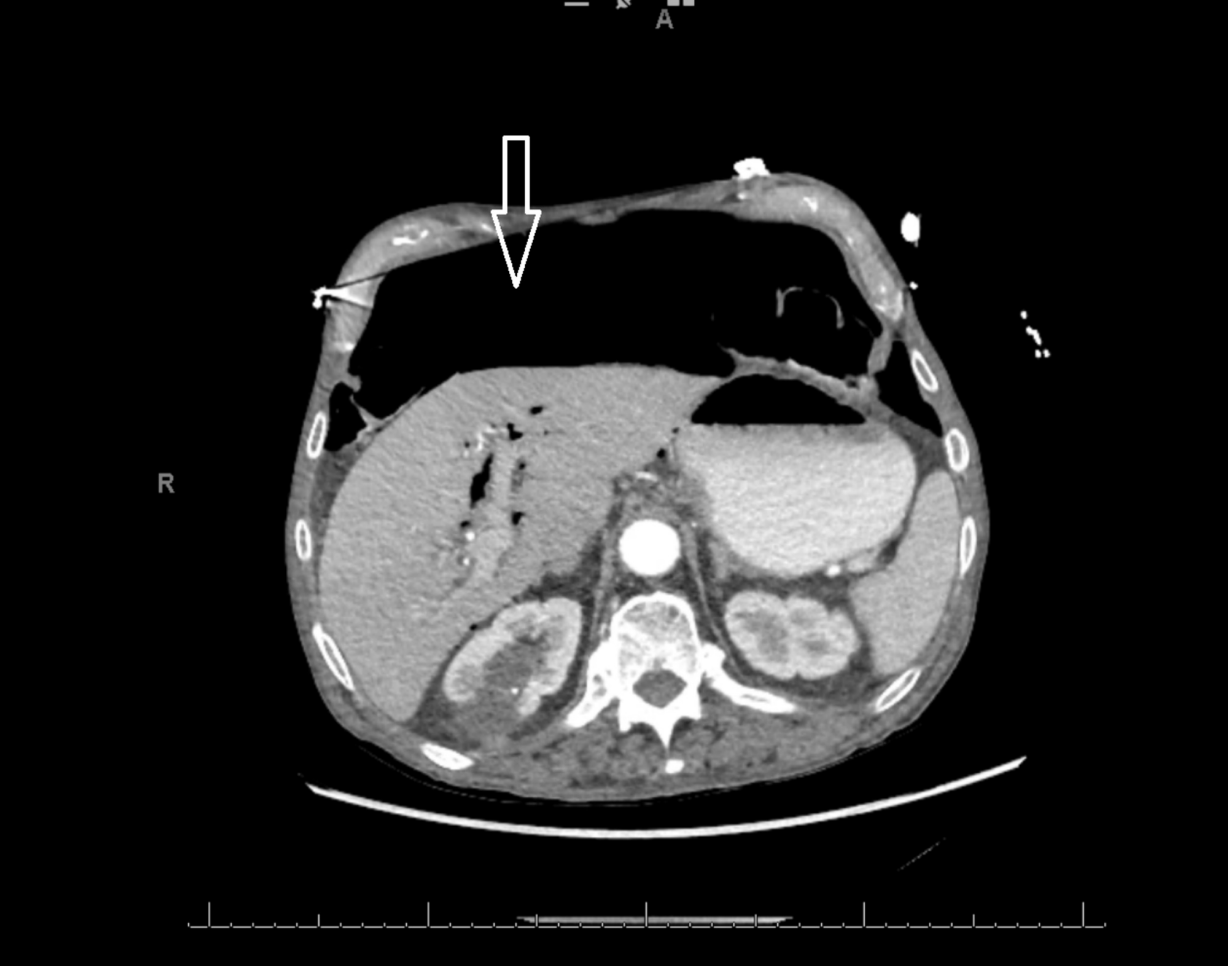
Axial CT scan of patient on admission showing free air in the peritoneal cavity (solid arrow) CT: computed tomography
